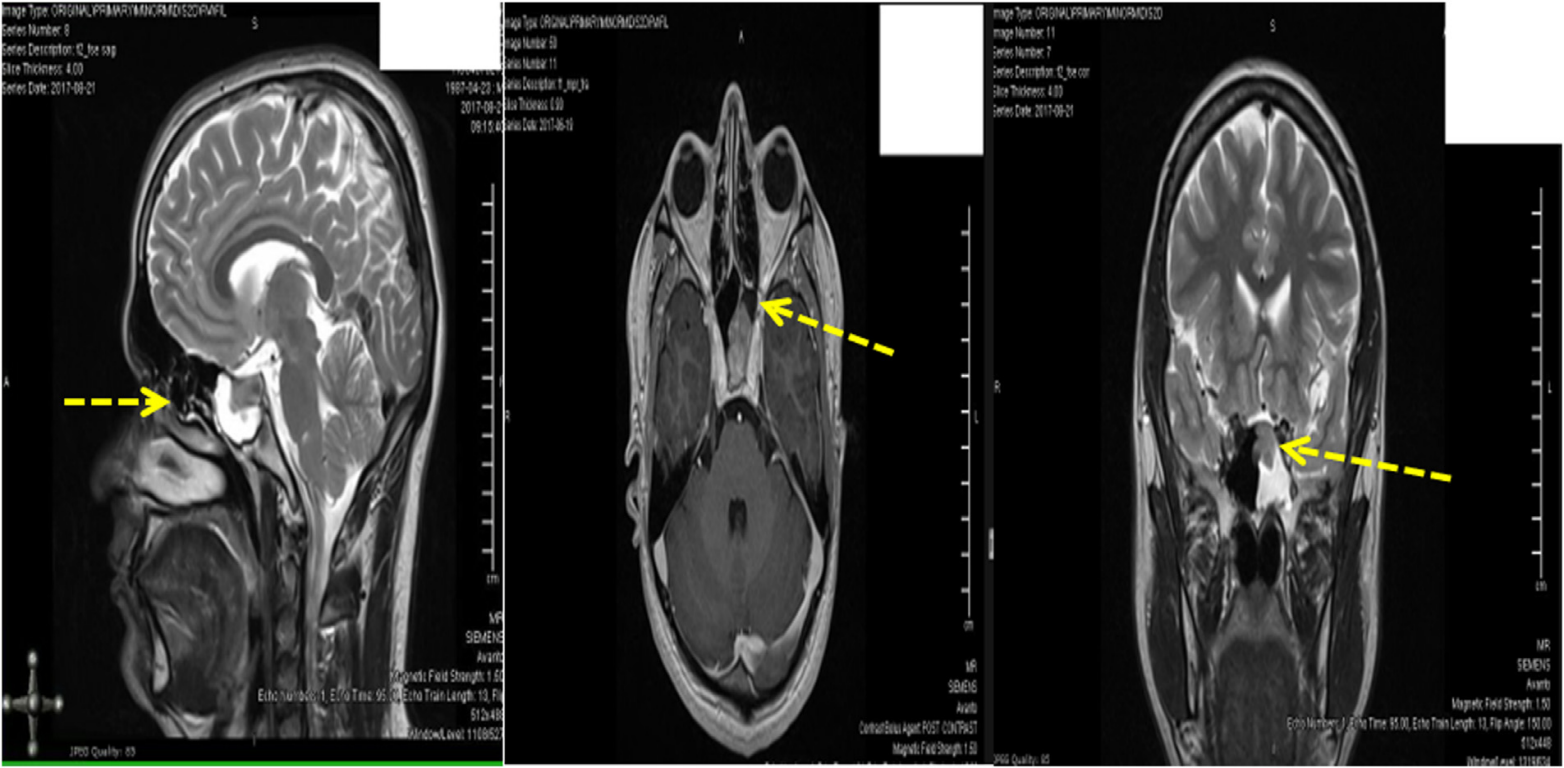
MRI HEAD showing large bony defect in the sellar floor with soft tissue in the anterior aspect of the sella with infrasellar extension into the left sphenoid sinus. (Dashed yellow arrow).
